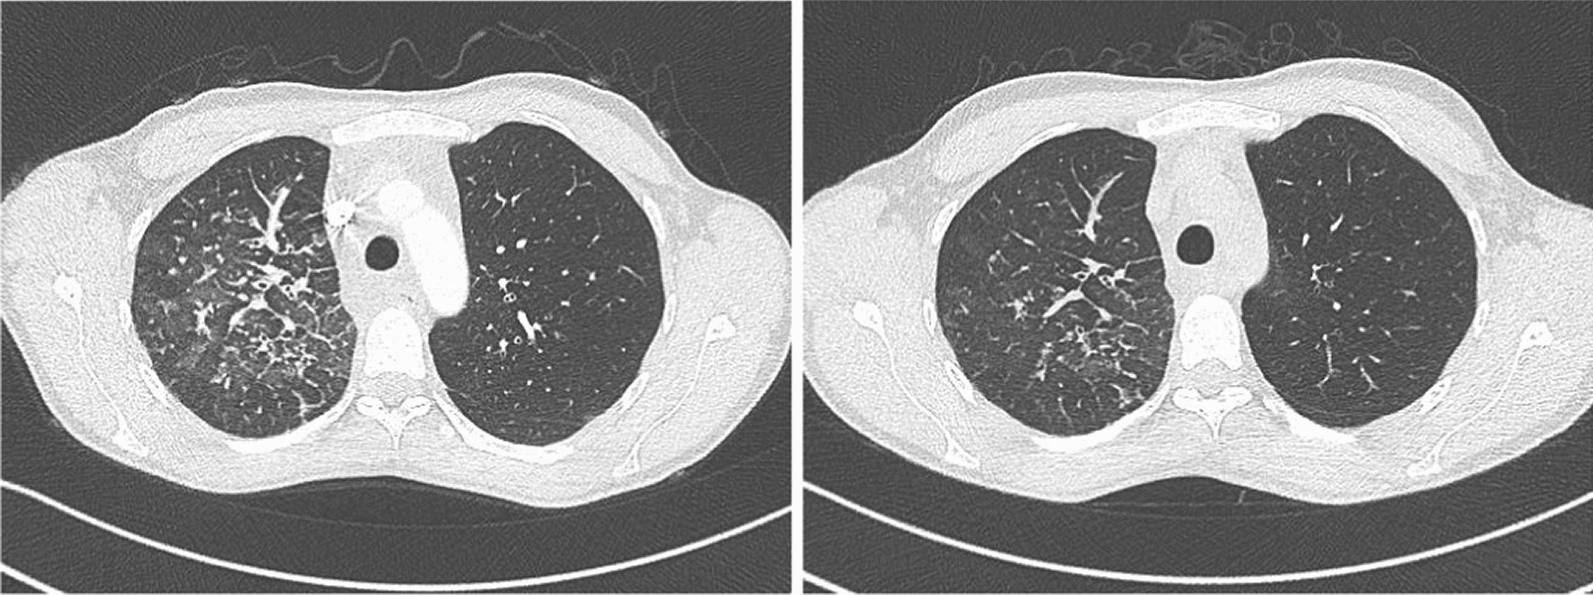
CT-Thorax before and after 3 months of treatment with sirolimus
